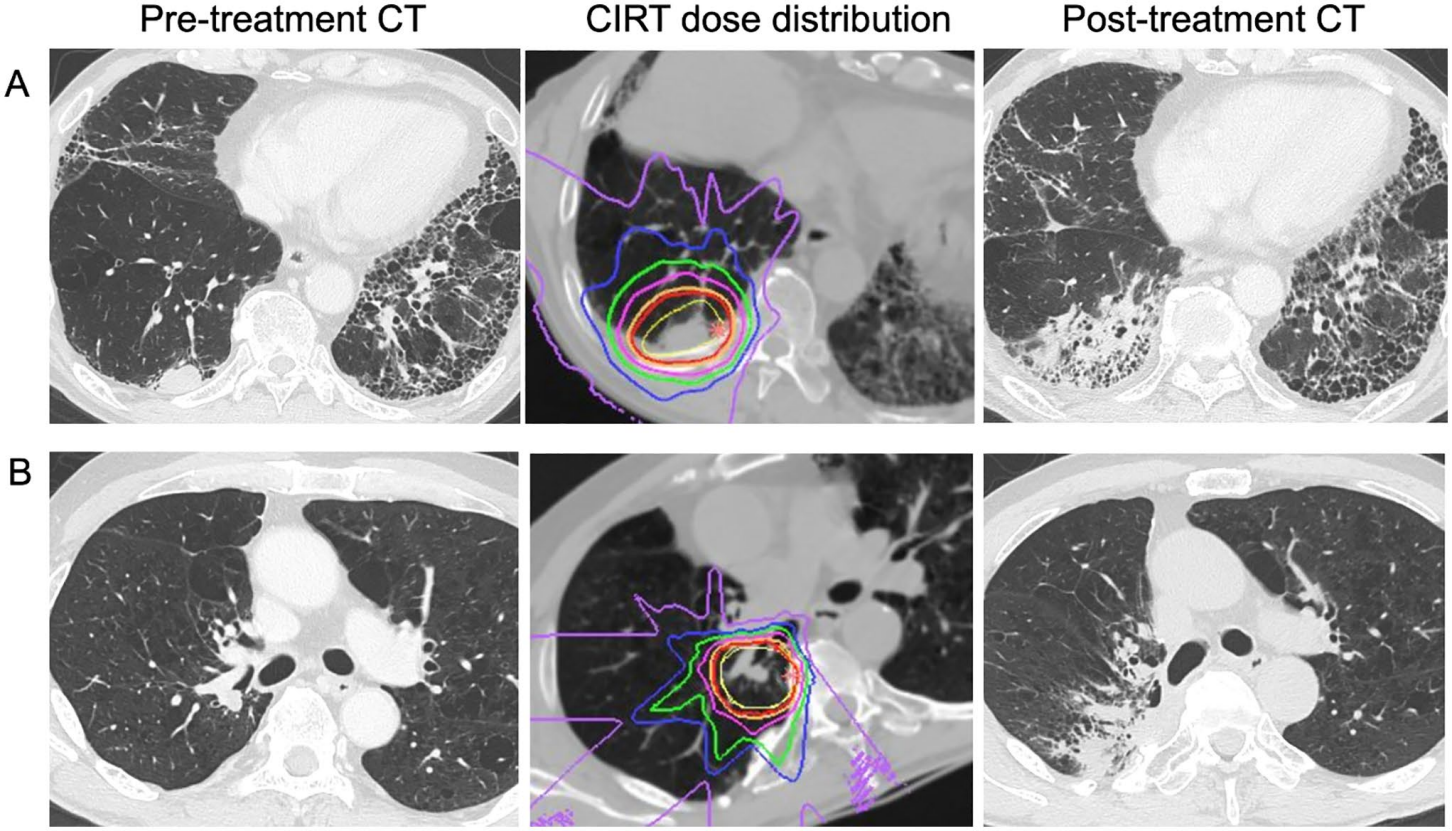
A Clinically diagnosed NSCLC (29 mm) with ANCA-associated vasculitis and interstitial pneumonia, receiving with 7.5 mg/day of prednisone (pre-treatment CT, left). The dose fractionation was 50 Gy (RBE) in a single fraction (CIRT dose distribution, middle). The red, yellow, pink, green, blue, and purple lines show 95%, 90%, 70%, 50%, 30%, and 10% doses, respectively; CTV is drawn with the thin yellow line. Radiation pneumonitis was limited to grade 1 (post-treatment CT image, right). B Right lower lobe adenocarcinoma (11 mm) with previous right upper-lobe resection (large cell carcinoma, pT2bN0M0) treated with carbon-ion radiotherapy with 68 Gy (RBE) in 12 fractions (protocol for central tumors), requiring treatment for grade 2 pneumonitis. *CT* computed tomography; *CIRT* carbon-ion beam radiotherapy; *NSCLC* non-small-cell lung cancer; *RBE* relative biological effectiveness; CTV ANCA
